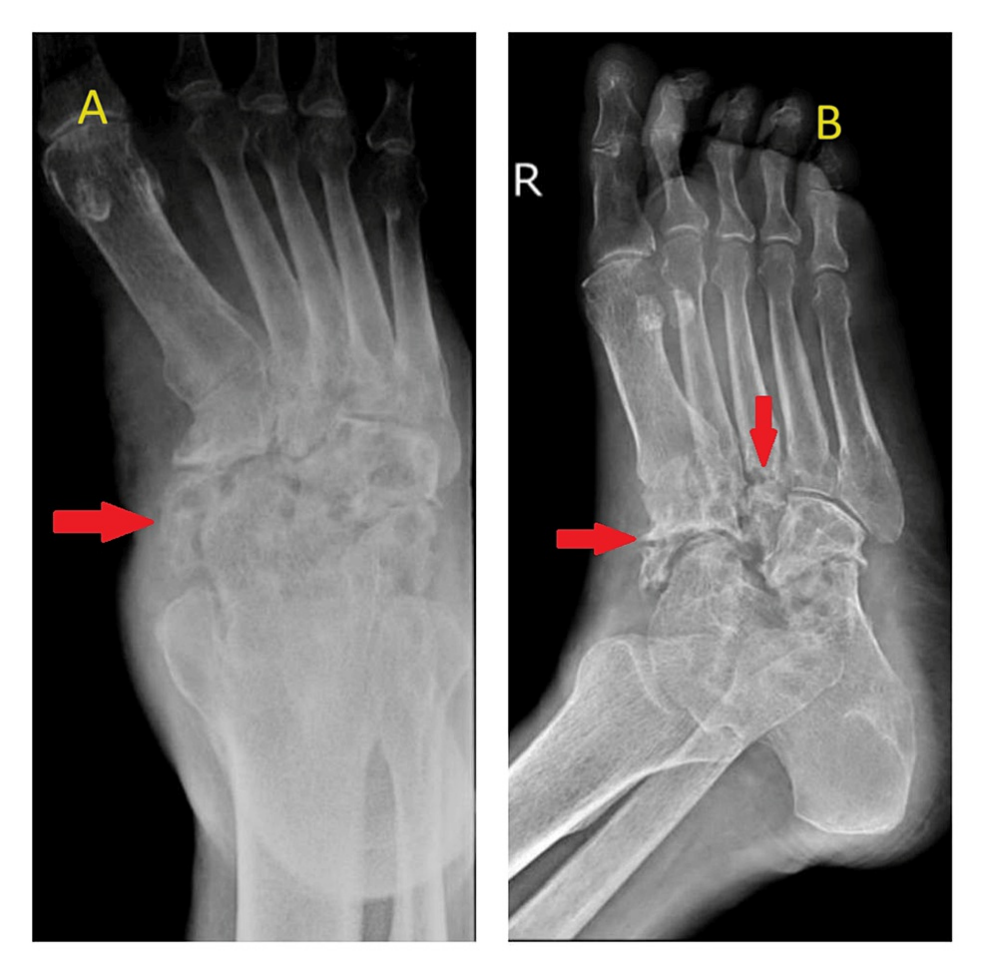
X-ray of right foot anteroposterior (A) and oblique (B) views showing lytic lesions noted in the tarsal bones. No bony erosion or destruction was noted. The joint space appeared normal. No other osteomyelitis changes were seen.
